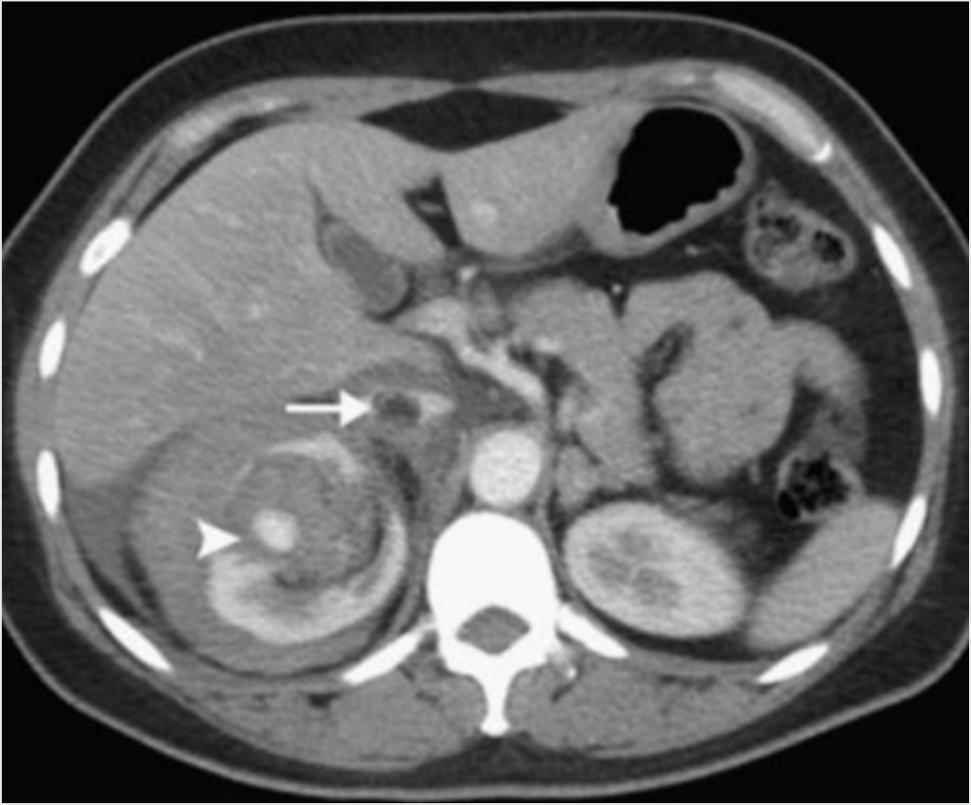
Contrast-enhanced CT showing two features: a perirenal hematoma and enhancing lesion with aneurysm formation (arrowhead) and a thrombus in the IVC (arrow) [15].
